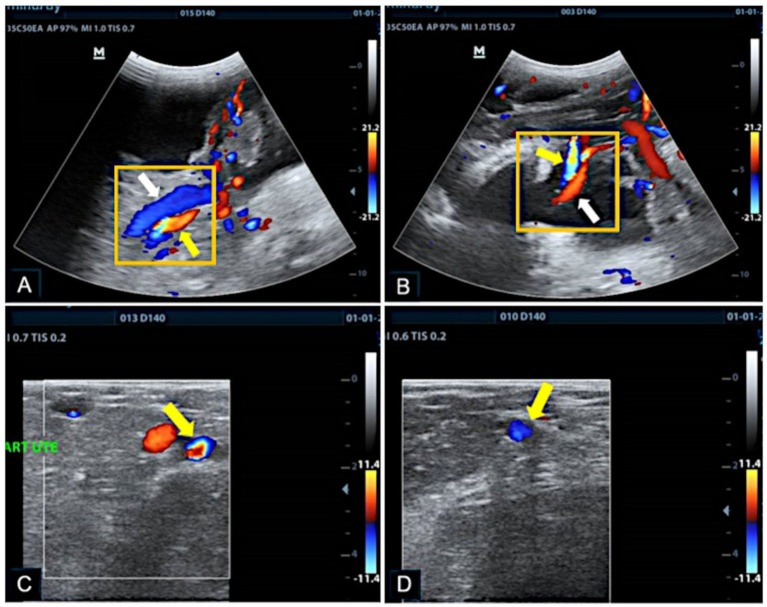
Color Doppler ultrasound image showing the umbilical (A,B) artery (yellow arrow), vein (white arrow), and uterine artery (C,D) at 140 days of pregnancy, in baseline TMR diet (BSTMR), (A,C) and TMR diet supplied with rumen-unprotected guanidinoacetic acid (GATMR) (B,D).
